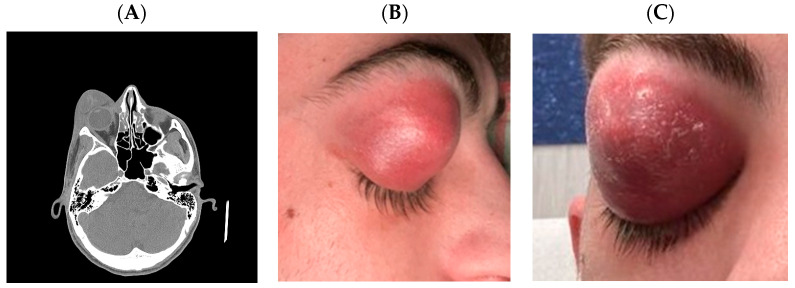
Right orbital cellulitis with periorbital abscess, proptosis, and associated sinusitis. (A) Head computed tomography scan that revealed a right orbital cellulitis with a large exophytic formation in the orbital and periorbital area inferiorly displacing the eyeball, together with right maxillary, frontal and ethmoidal sinusitis. (B,C) Patient’s pictures showing marked right periorbital edema, erythema and violaceous swelling causing complete mechanical ptosis and proptosis. The lesion appeared fluctuant, consistent with a subcutaneous abscess.
